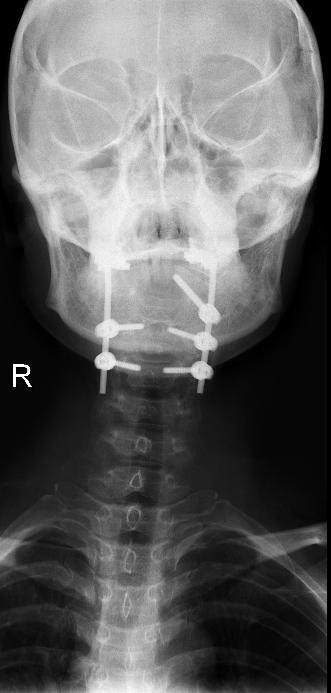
Anteroposterior X-ray postoperative image.
